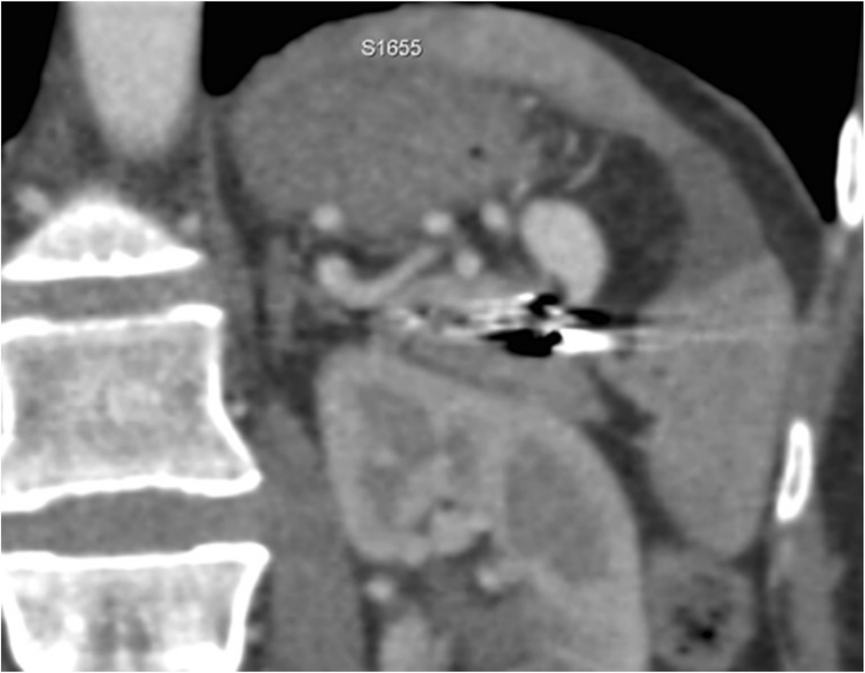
Computed tomography scan (coronal view) revealed the reperfusion of the aneurysmal sac.
